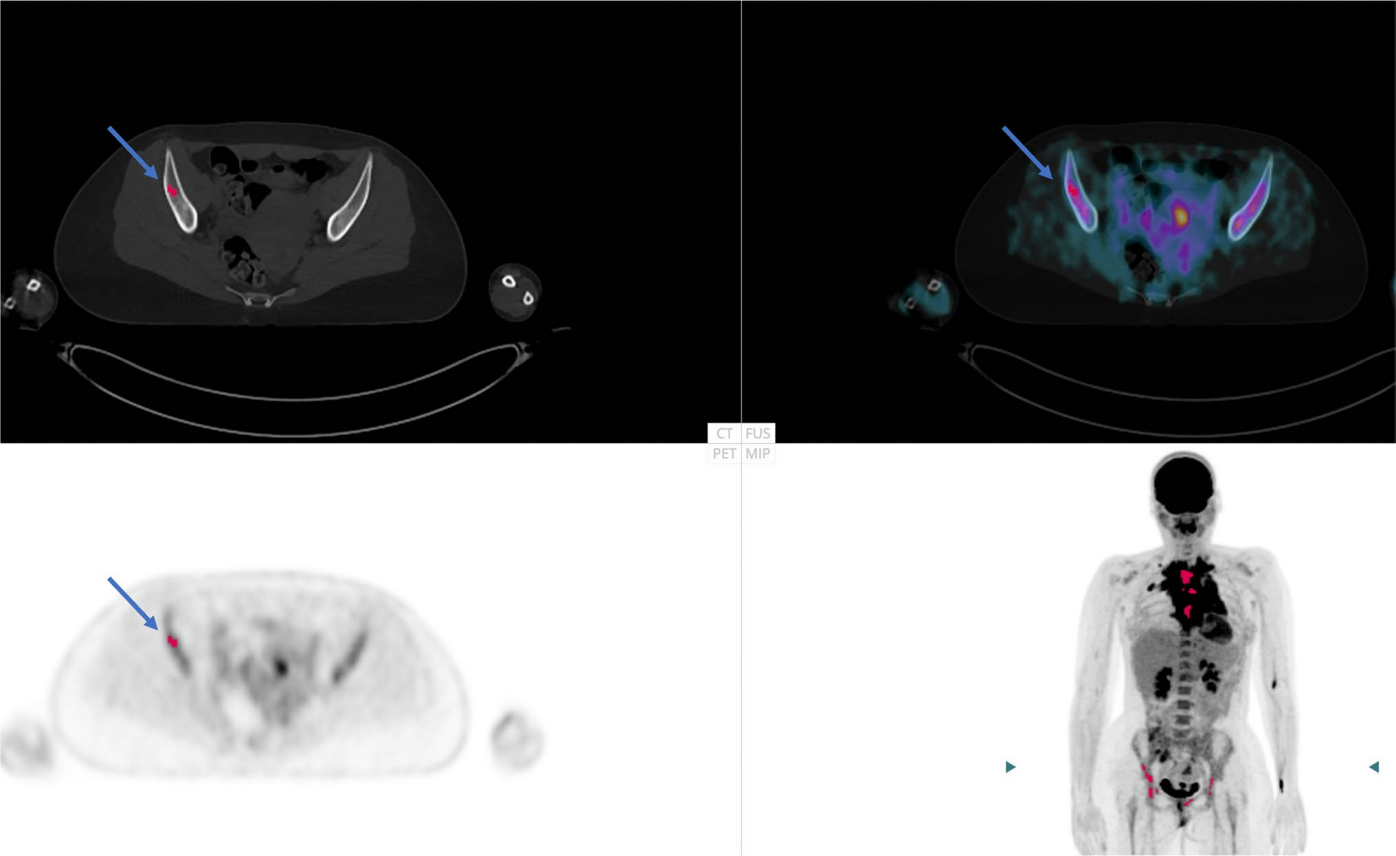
Example of a patient with focal skeletal/bone marrow uptake (highlighted with a red dot by artificial intelligence (AI), blue arrow) and increased diffuse bone marrow uptake (SUV_index_ 1.4). In this patient, 7 of the 10 physicians agreed with AI regarding focal bone marrow involvement
